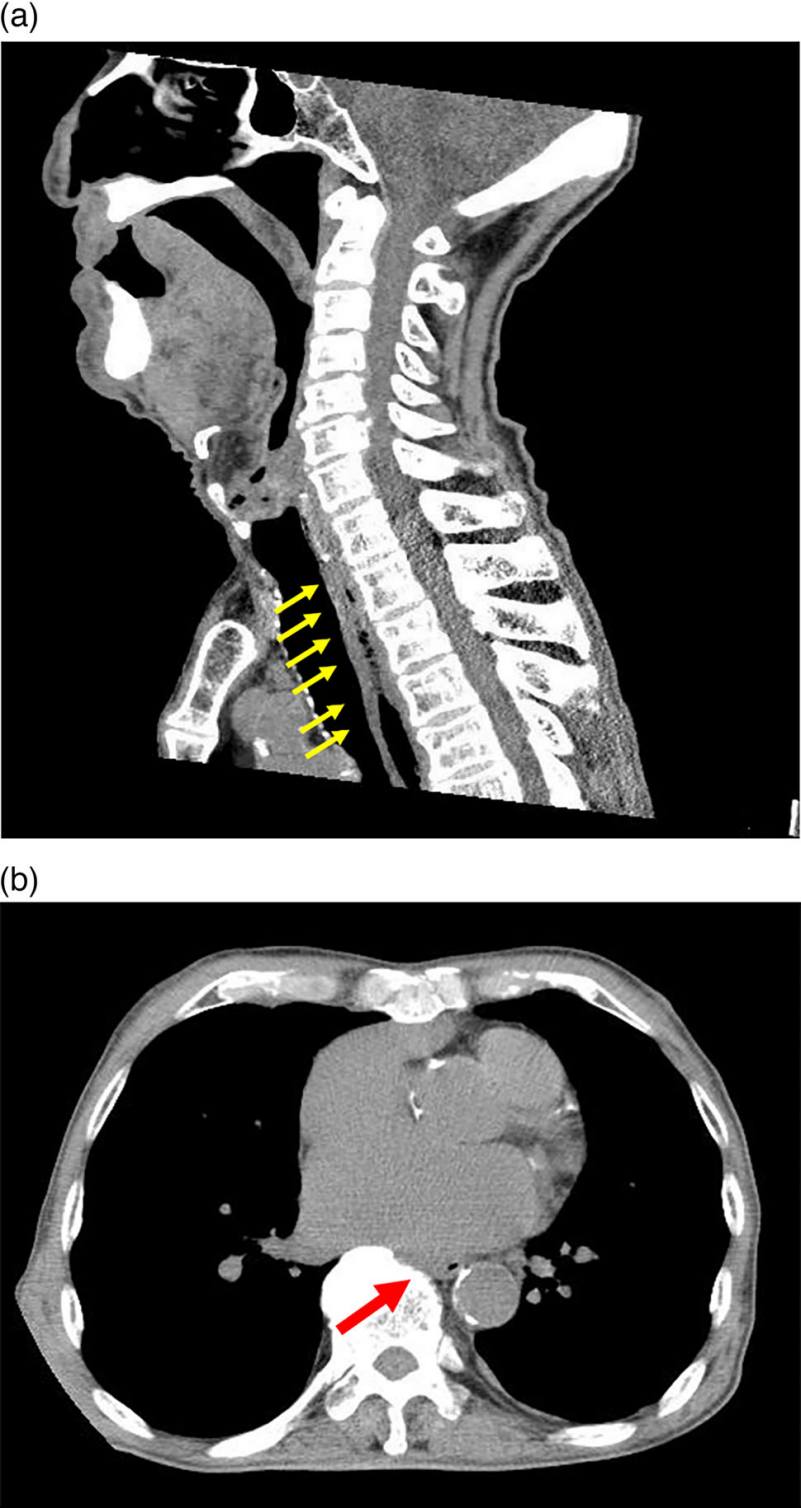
Sagittal computed tomography reveals thickening of the entire esophageal wall with an upper esophageal predominance (a). Horizontal computed tomography shows compression of the esophagus by the left atrium (b).
